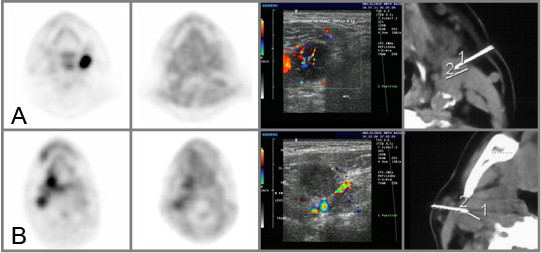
From left to right: transaxial FDG PET-, transaxial FMISO PET-, CDS- and transaxial CT scans in two different patients. A:with a normoxic tumor (pO_2 _≤ 2.5 mmHg = 13.0%, pO_2 _≤ 5.0 mmHg = 15.1%, pO_2 _≤ 10.0 mmHg = 17.0%, median pO_2 _= 36.2 mmHg, mean pO_2 _= 41.1 mmHg; CPD = 12.05%; FDG_SUVmax _= 17.84, FDG_SUVmean _= 14.80; FMISO_T/M _= 1.31, FMISO_T/B _= 1.07). B:with a hypoxic tumorB (pO_2 _≤ 2.5 mmHg = 37.7%, pO_2 _≤ 5.0 mmHg = 48.8%, pO_2 _≤ 10.0 mmHg = 58.1%, median pO_2 _= 5.5 mmHg, mean pO_2 _= 17.7 mmHg; CPD = 2.71%; FDG_SUVmax _= 9.34, FDG_SUVmean _= 8.00; FMISO_T/M _= 1.60, FMISO_T/B _= 0.88). 1/2 = distance measurements for the guidance of the polarographic needle electrode
